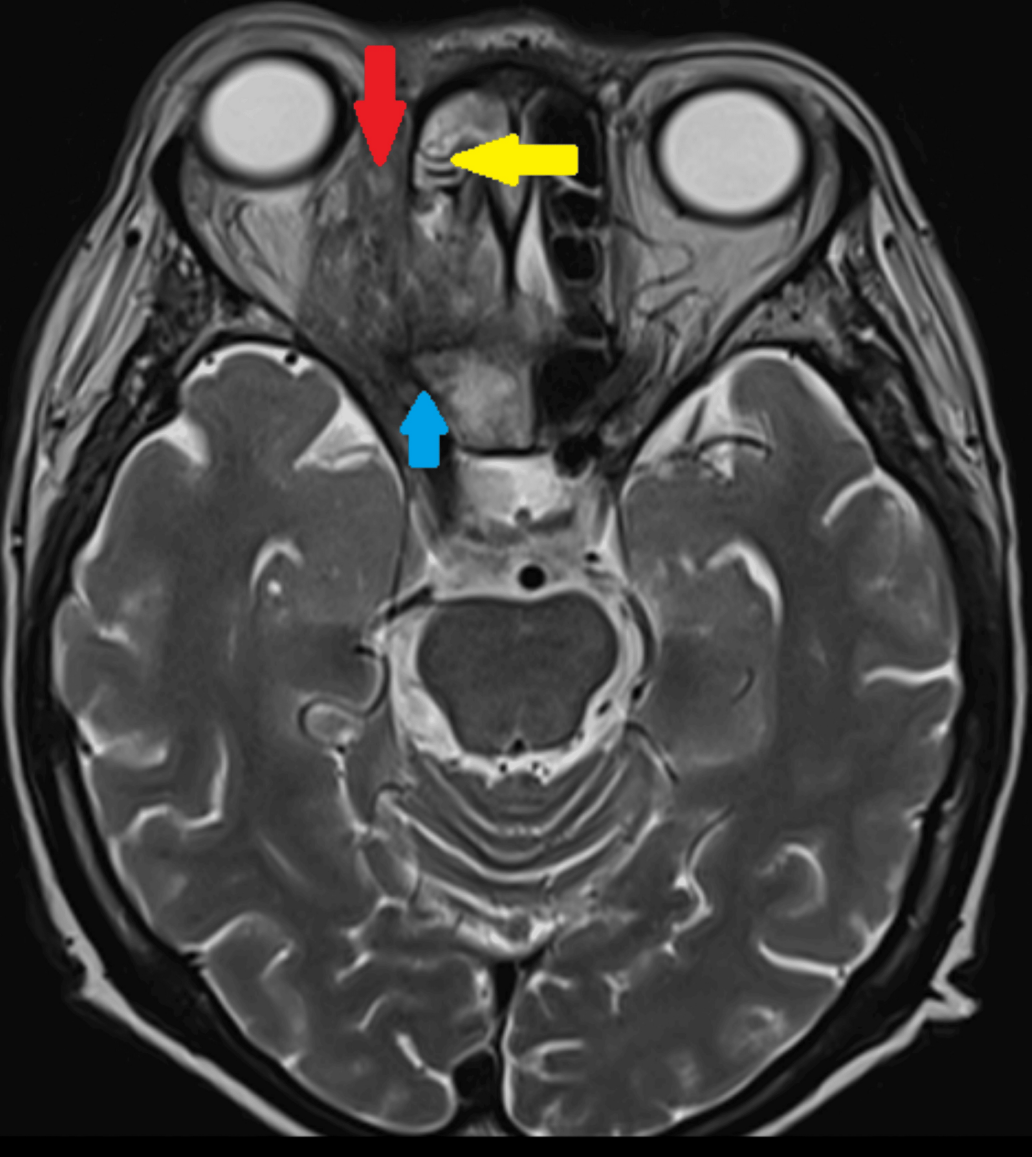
MRI showing hypointense lesion in right ethmoid sinus (yellow arrow) with right eye proptosis and subperiosteal collection at the medial wall of right orbit extending into extraconal compartment (red arrow). Enhancement surrounding optic nerve with extension into orbital apex with dural enhancement of anteroinferior part of right cavernous sinus (blue arrow).
